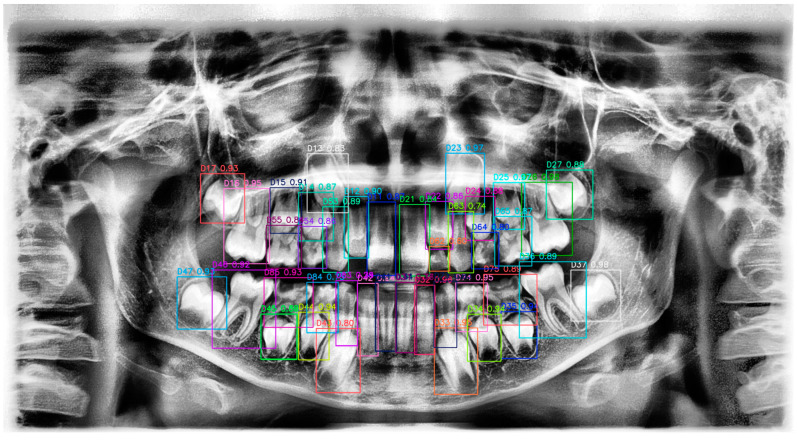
Detected teeth with confidence scores on panoramic radiograph using YOLOv10. This figure shows a panoramic radiograph with bounding boxes indicating the detected tooth regions, labeled by tooth identifiers and confidence scores. Each bounding box represents the YOLOv10’s prediction, with different colors used to distinguish individual teeth. The confidence score for each detection is displayed alongside the corresponding tooth label, indicating the model’s certainty in identifying each tooth. This visualization demonstrates the model’s capability in accurately localizing and classifying teeth within a complex radiographic image.
